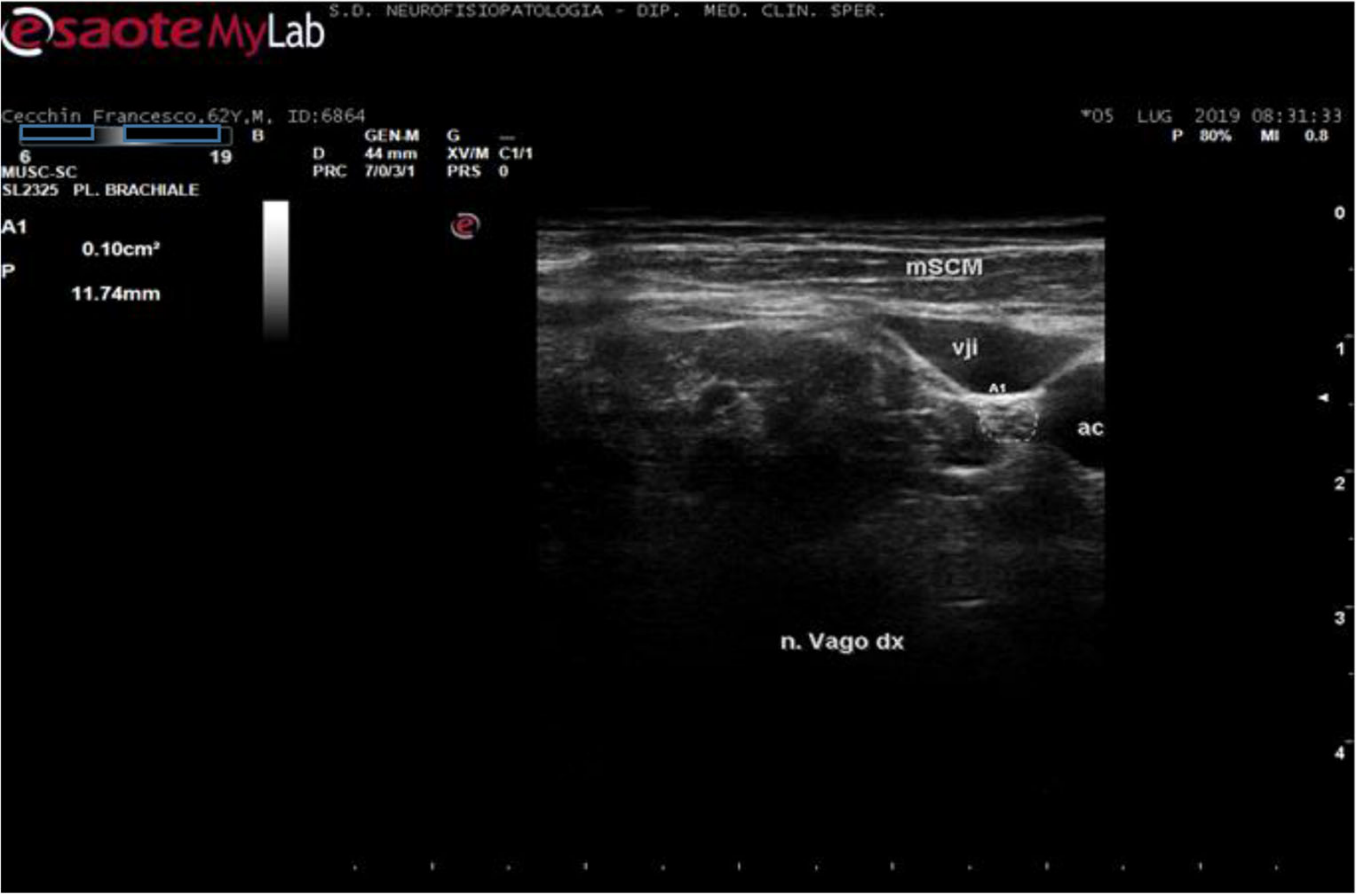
Typical axial high-resolution ultrasonography (HRUS) of short-axis view of the right VN (inside the dotted line) in a healthy control subject (C.F., M, 62 years). mSCM, sternocleidomastoid muscle; vji, internal jugular vein; ac, carotid artery; dx (destri), right side
